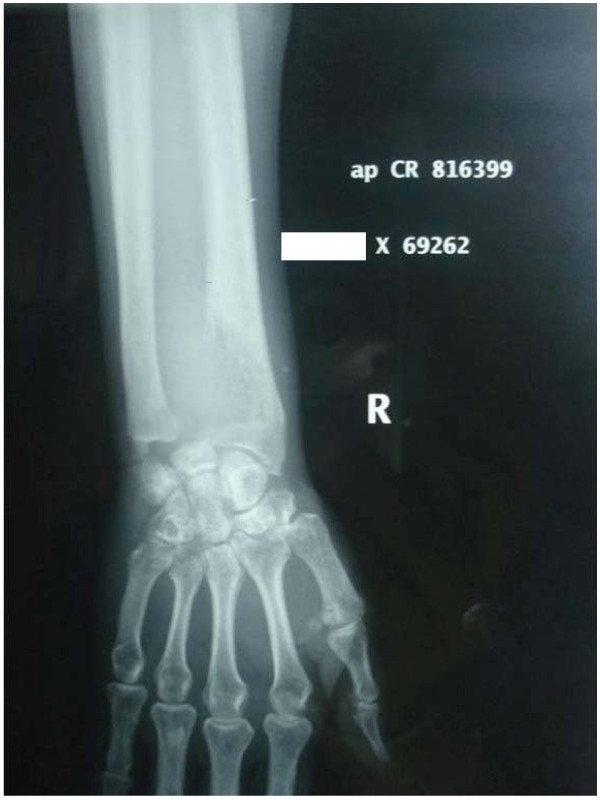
Preoperative radiograph showing GCT of the distal radius.
